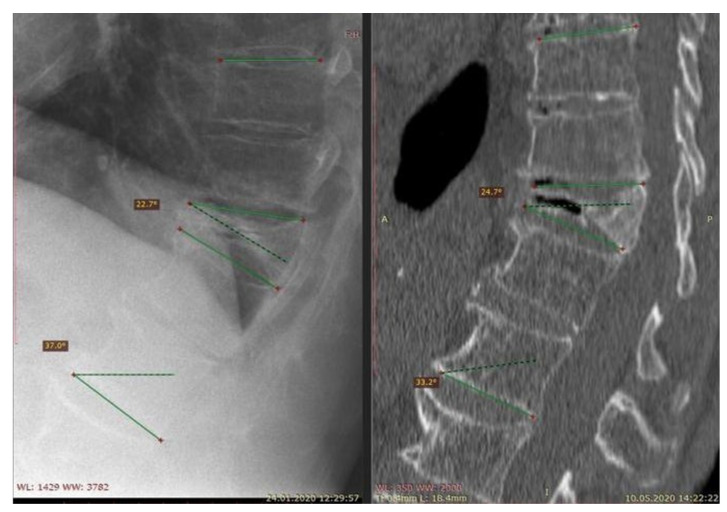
Images (from the left a X-ray and a CT scan respectively) of a vertebra injured from OVCF after conservative treatment failure.
